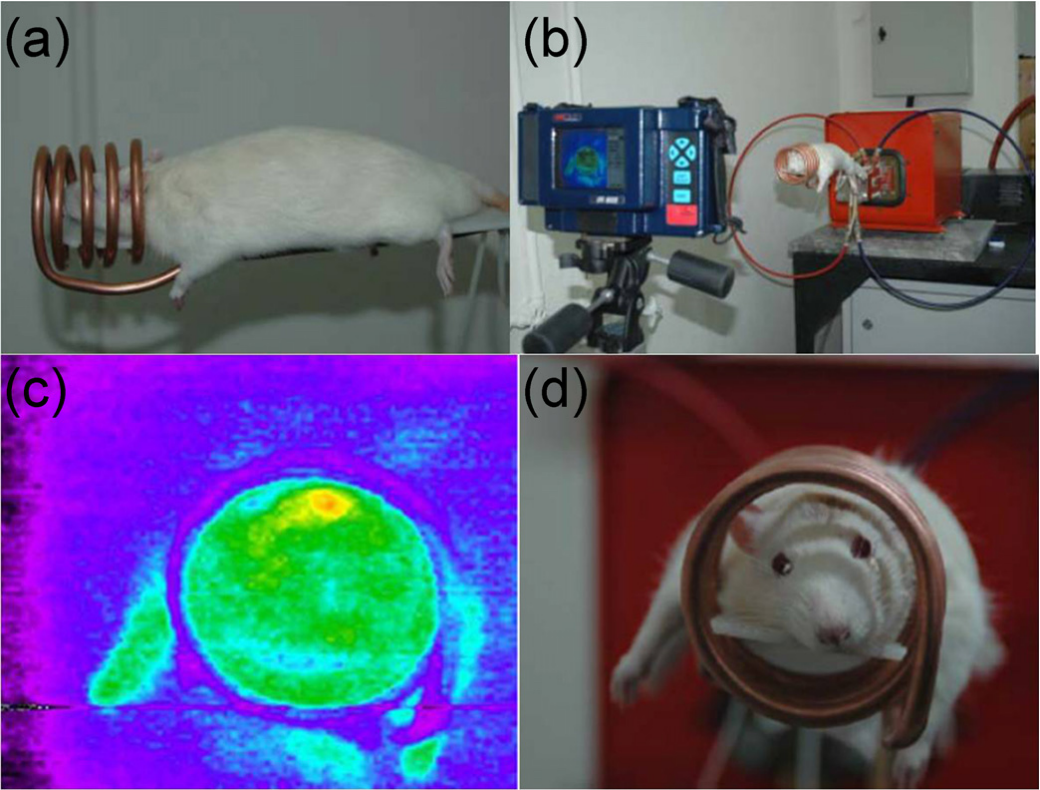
Ferrofluids containing IONPs are synthesized and characterized as possible agents for medical treatment and diagnosis. Specifically, novel iron-oxide-based NPs are investigated (i) as contrast agents for MRI, and (ii) for tumor treatment using the technique of magnetic hyperthermia where magnetic NPs are injected in the tumor and heated by applying a strong ac magnetic field. In the left picture the temperature increase of an extracranial tumor, after injecting a small quantity of ferrofluid and irradiating with low frequency radiofrequency waves (150 kHz).
